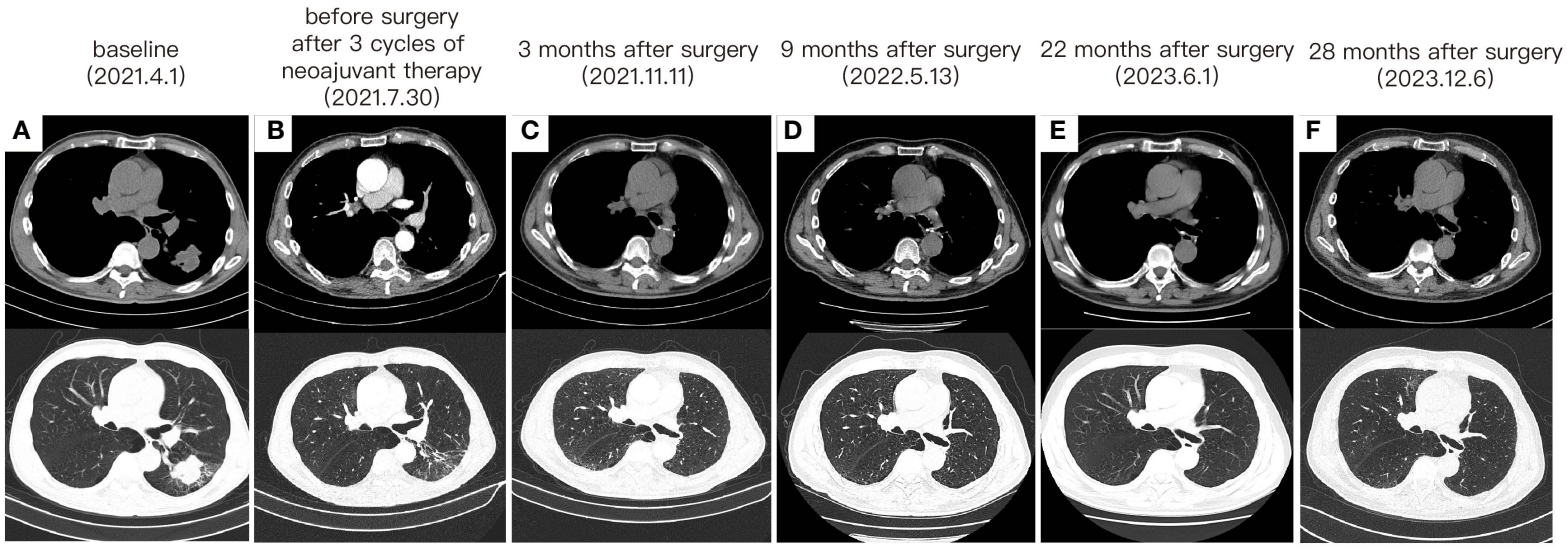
The change of tumor on CT imaging. (A) The CT images before any treatment. (B) The CT images after 3 cycles of neoadjuvant therapy and before surgery. (C) CT taken at 3 months after surgery. (D) CT taken at 9 months after surgery. (E) CT taken at 22 months after surgery. (F) CT taken at 28 months after surgery.
